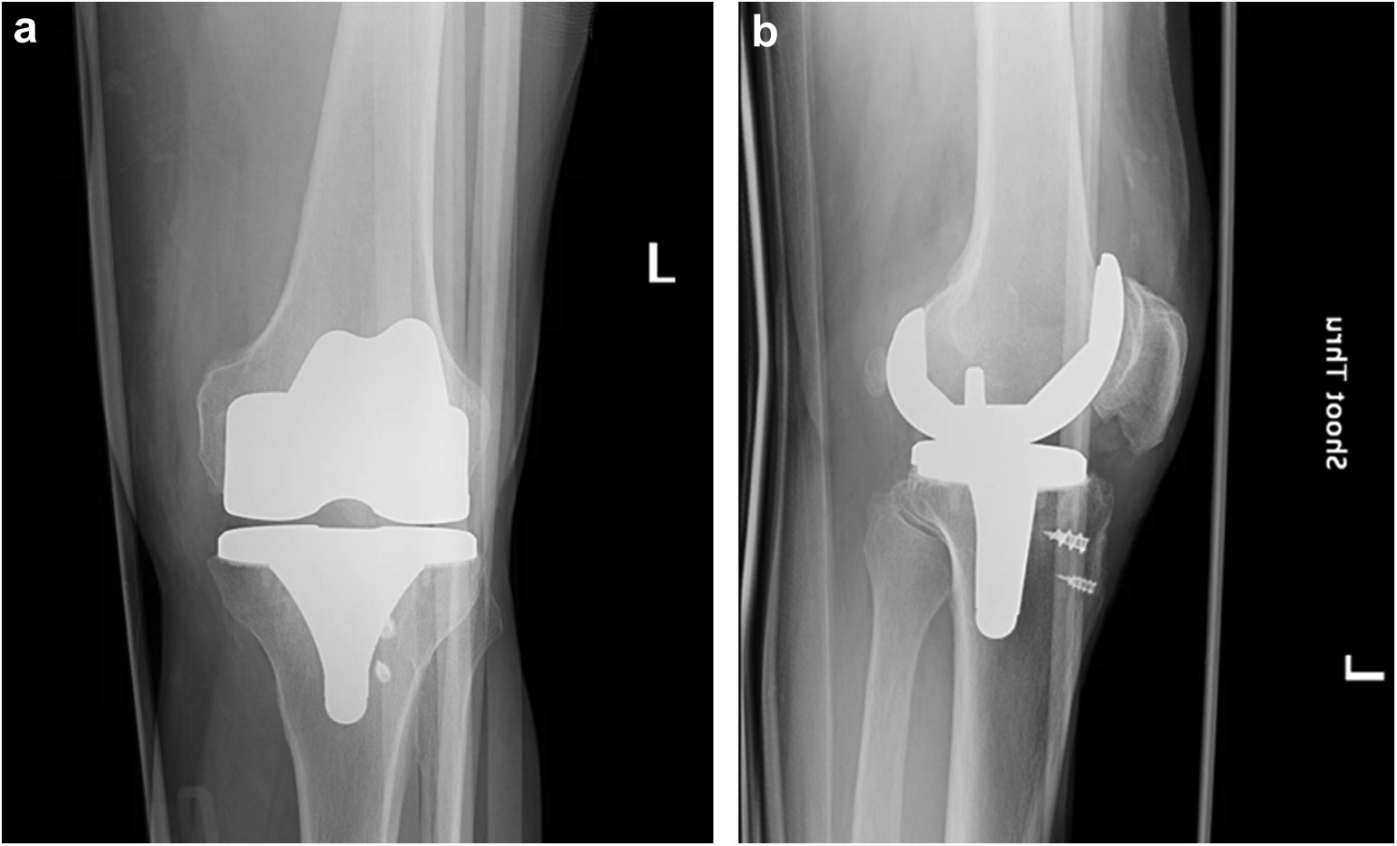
Anteroposterior (a) and lateral (b) radiographs demonstrating interval reduction of a left lateral TKA dislocation.
